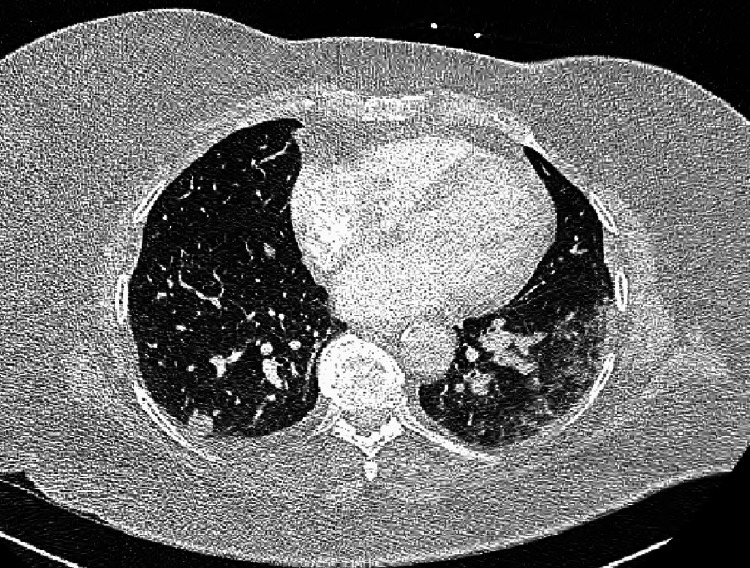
CT of the chest with contrast showing multiple bilateral pulmonary nodules
